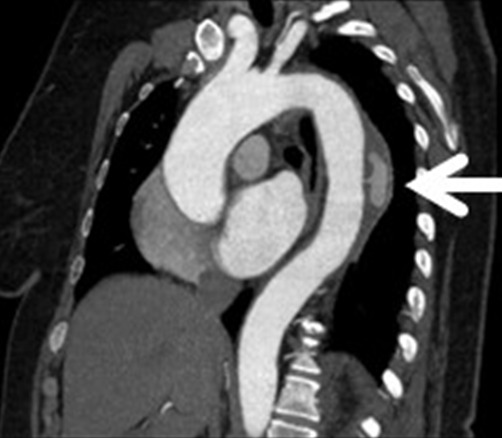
Enhaced CT scan 3 months later: ectasic aorta with stable hematoma
